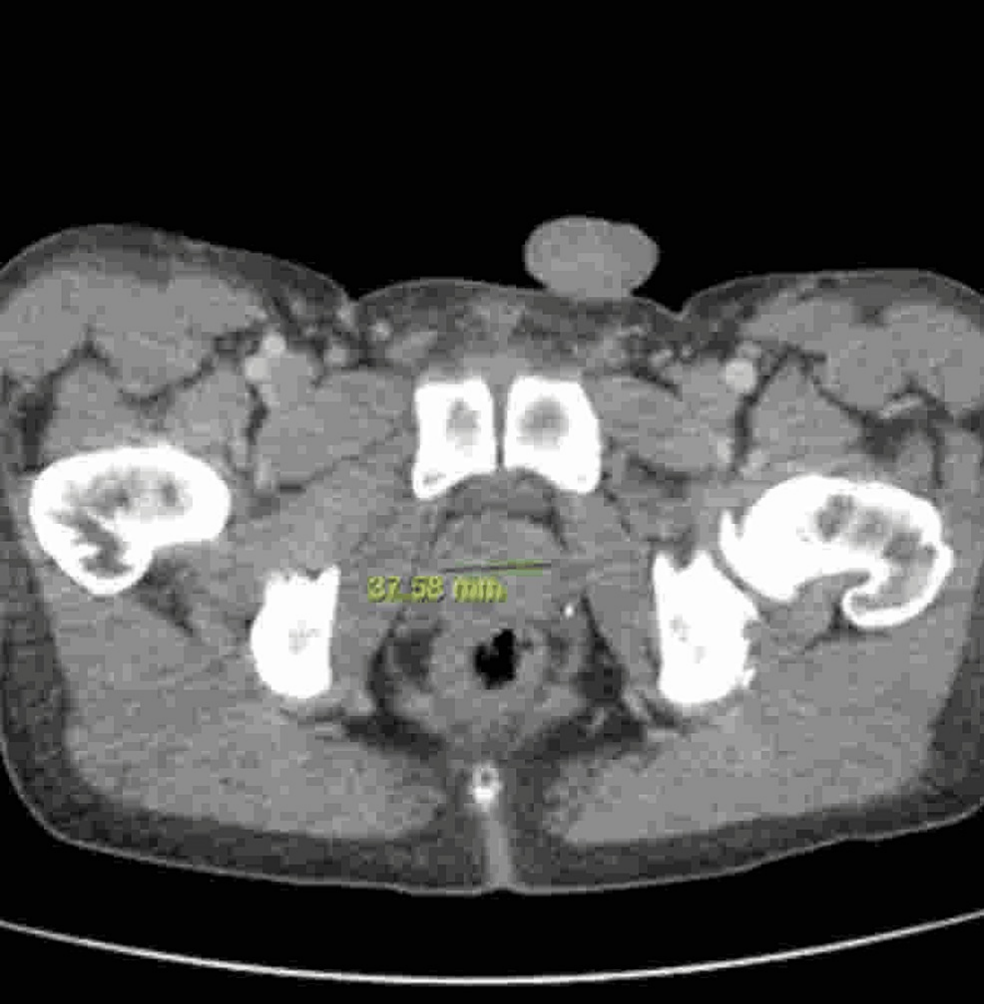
CT abdomen and pelvis (axial view) with contrast The image shows mild-moderate focal concentric thickening of the rectal wall, a hypodense lesion in the left hepatic lobe (1.7 cm), and a posterior perirectal mesenteric nodule (1.4 cm) CT: computed tomography
